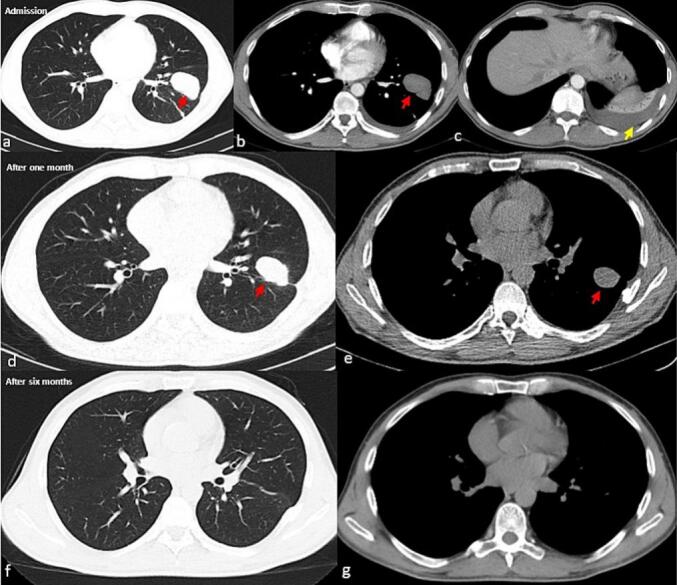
The patient chest CT scans without contrast at admission, one month after admission, and six months after admission. Figures a, d, and f show the parenchymal view, while figures b, c, e, and g present the mediastinal view of the patient's chest CTs. The imaging reveals the spontaneous resolution of traumatic pulmonary pseudocyst (red arrow) and the complication of pseudocyst with hemothorax (yellow arrow).
